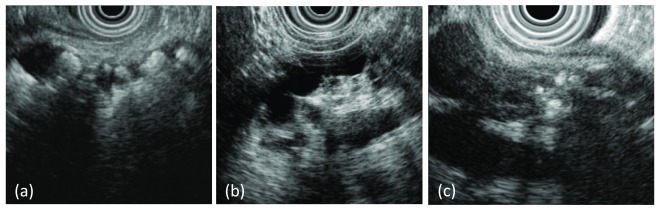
Endoscopic ultrasound images characterizing features of chronic pancreatitis. (a) Anechoic tubular structure with multiple hyperechoic structures with shadowing. Depicts a dilated main pancreatic duct with multiple calcified stones. (b) Anechoic tubular structure depicting dilated, irregular main pancreatic duct. (c) Diffuse echogenicity with hyperechoic foci with stranding. Depicts pancreatic lobularity with calcifications.
